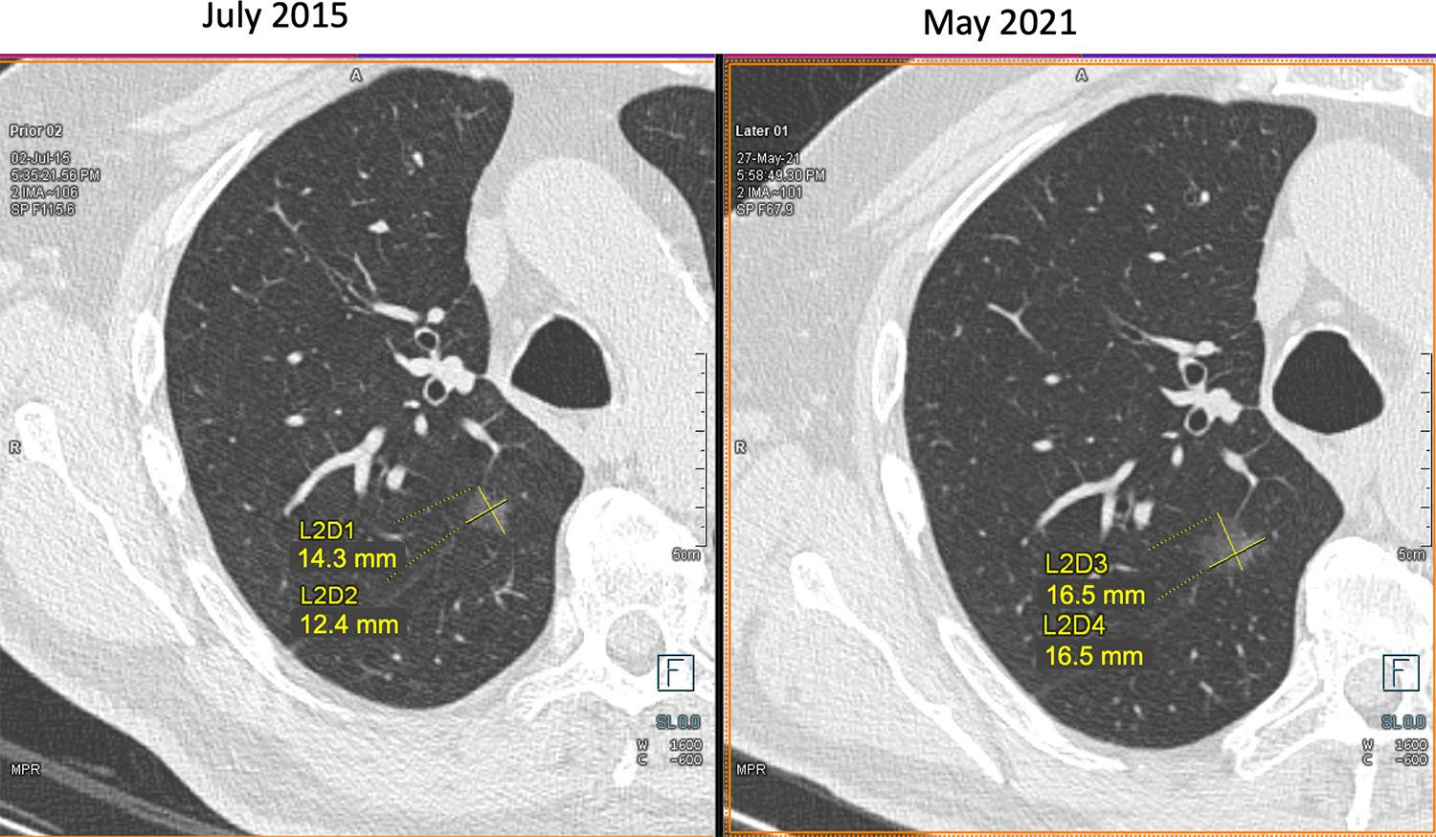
A–B. Measurement of a non-solid nodule (ground glass opacity) in the apical segment of the right lower lobe and its growth. Axial CT image showing a non-solid nodule in apical segment of the right lower lobe. The measurement by manual caliper at first detection (maximum diameter 14.3 mm, orthogonal diameter 12.4 mm, mean diameter 13.4 mm) and follow up scan (B: 16.5 × 16.5 mm, mean diameter 16.5 mm). The variable and limited density difference between the non-solid nodule and the surrounding parenchyma represents a factor for variability of semi-automated volume segmentation
